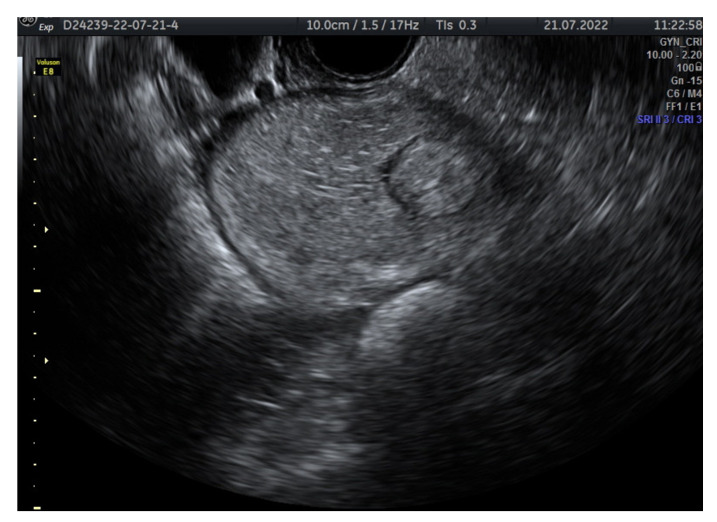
Transvaginal ultrasound of a mature teratoma. Echogenic foci or multiple hyperechoic lines are due to hairs floating.
